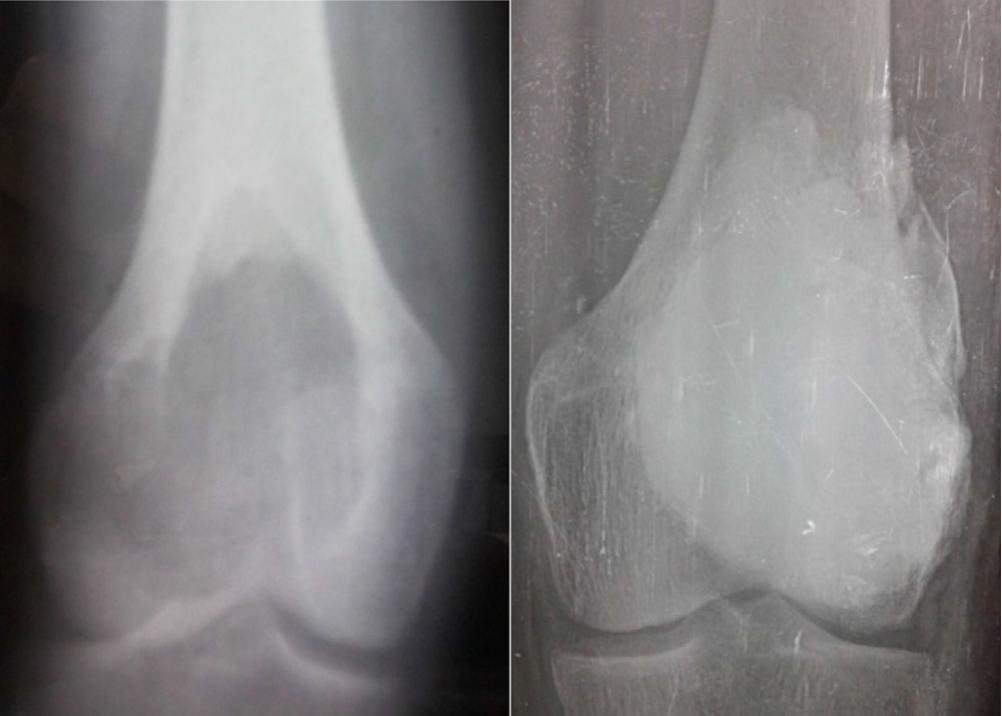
Serial plain radiographs (A) 18 years old patient with GCT in the distal femur (case 3), (B) 30 months last follow-up after endoscopic curettage and percutaneous bone cement application with no radiologic evidence of recurrence.
